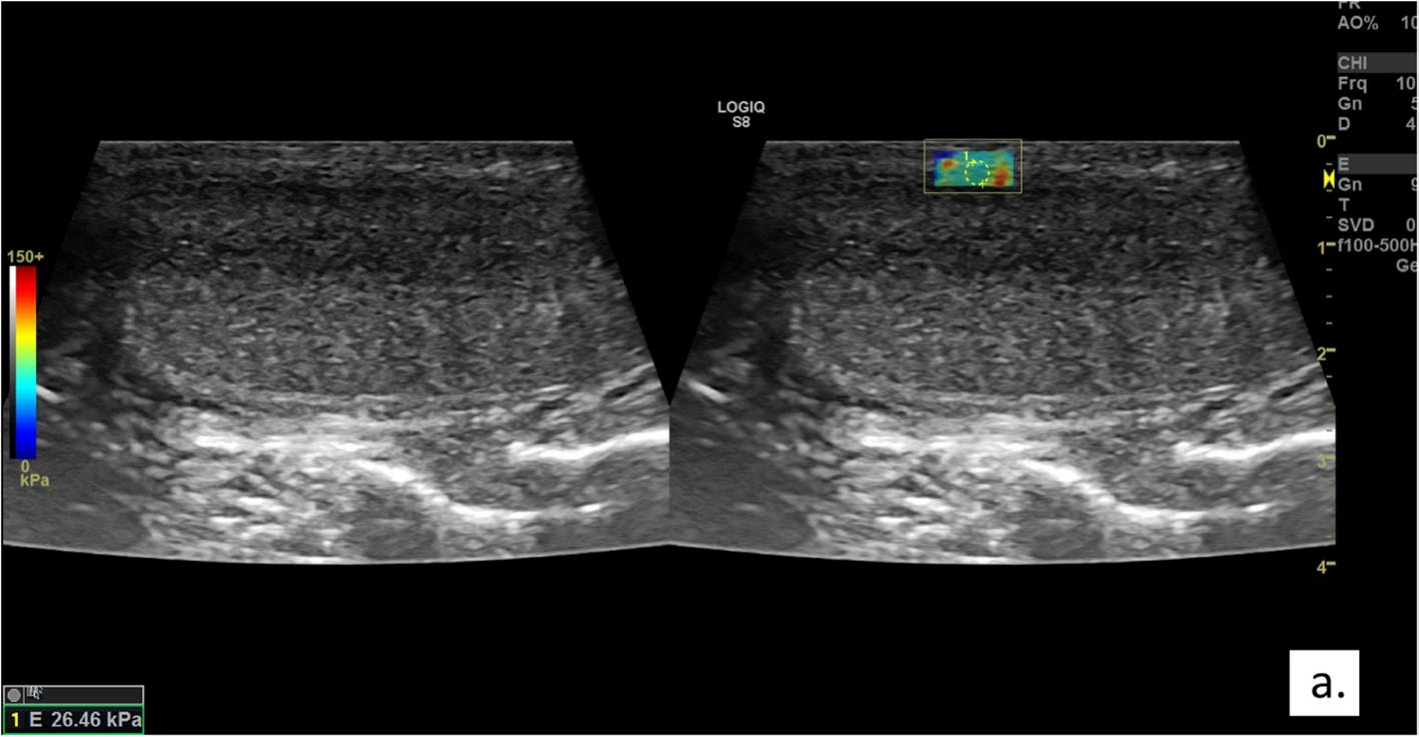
Example of a shear wave elastography image of Tunica Albuginea (TA) including quantitative measurement data expressed in kilopascal (kPa) in a patient with Peyronie's Disease (PD) (case group). The elastosonography mode simultaneously provides two images of the same area: The left image is in US—B—mode while on the right image is simultaneously displayed for stiffness measurement. The yellow box indicates the region of interest (ROI) observed in the shear wave elastography image, and the yellow circles indicate the intensity of the stiffness expressed values in kPa. In addition, the software provides a colorimetry map of the stiffness of the ROI. In particular, the red color indicates high stiffness while the blue color indicates lower stiffness. TA: tunica albuginea; kPa: kilopascal; PD: Peyronie’s Disease US: ultrasound; ROI: region of interest
